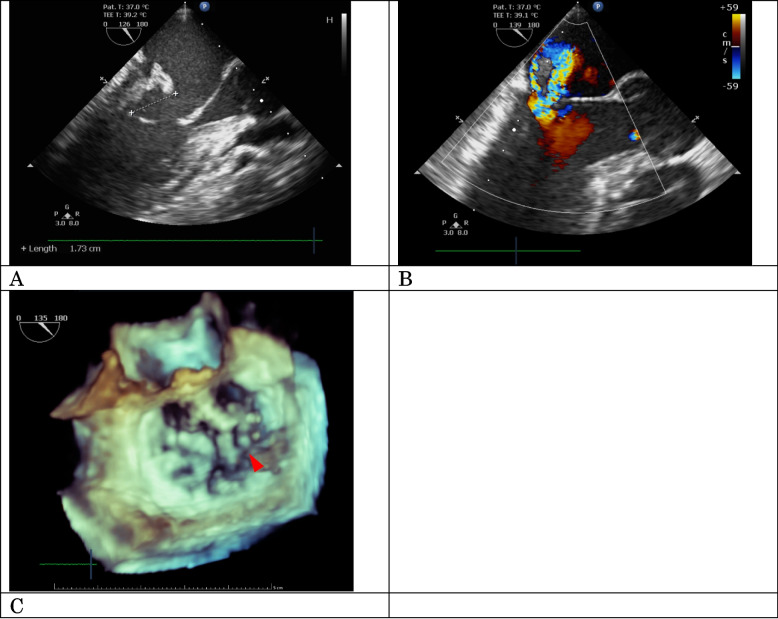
Preoperative echocardiographic findings. Echocardiography showed a 17-mm mobile mass at the posteromedial commissure mitral valve (A) and associated severe mitral regurgitation (B). Three-dimensional echocardiography revealed vegetation (arrow) on the mitral valve’s posteromedial site (C)
